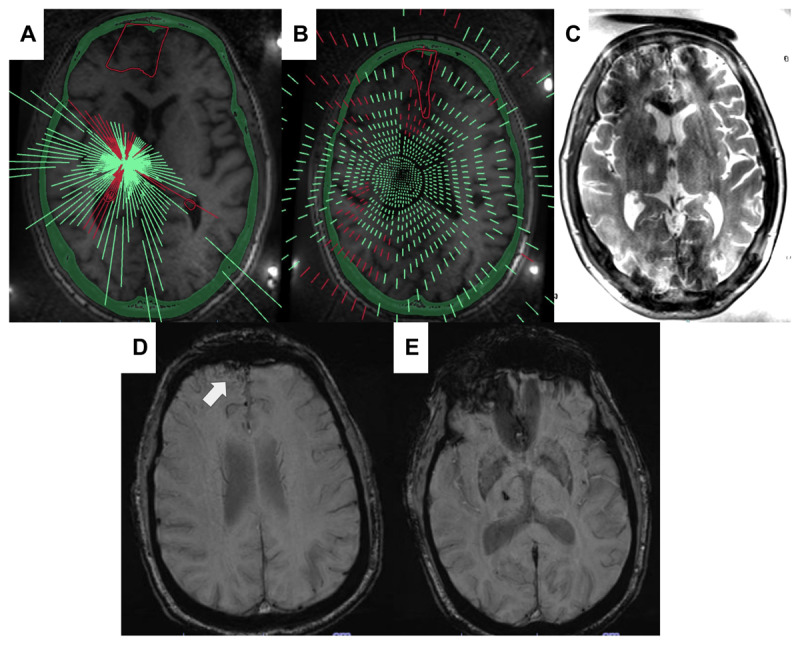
A and B depicts the procedural demonstration of transducer element summaries with the no pass zones of the AVM outlined in red. C depicts the subsequent postoperative T2-weighted axial MRI demonstrating the thalamotomy lesion in the right thalamus. Images D and E are post-procedural susceptibility weighted imaging demonstrating an unchanged right frontal AVM (D) and the right thalamotomy (E), with no additional evidence of hemorrhage.
